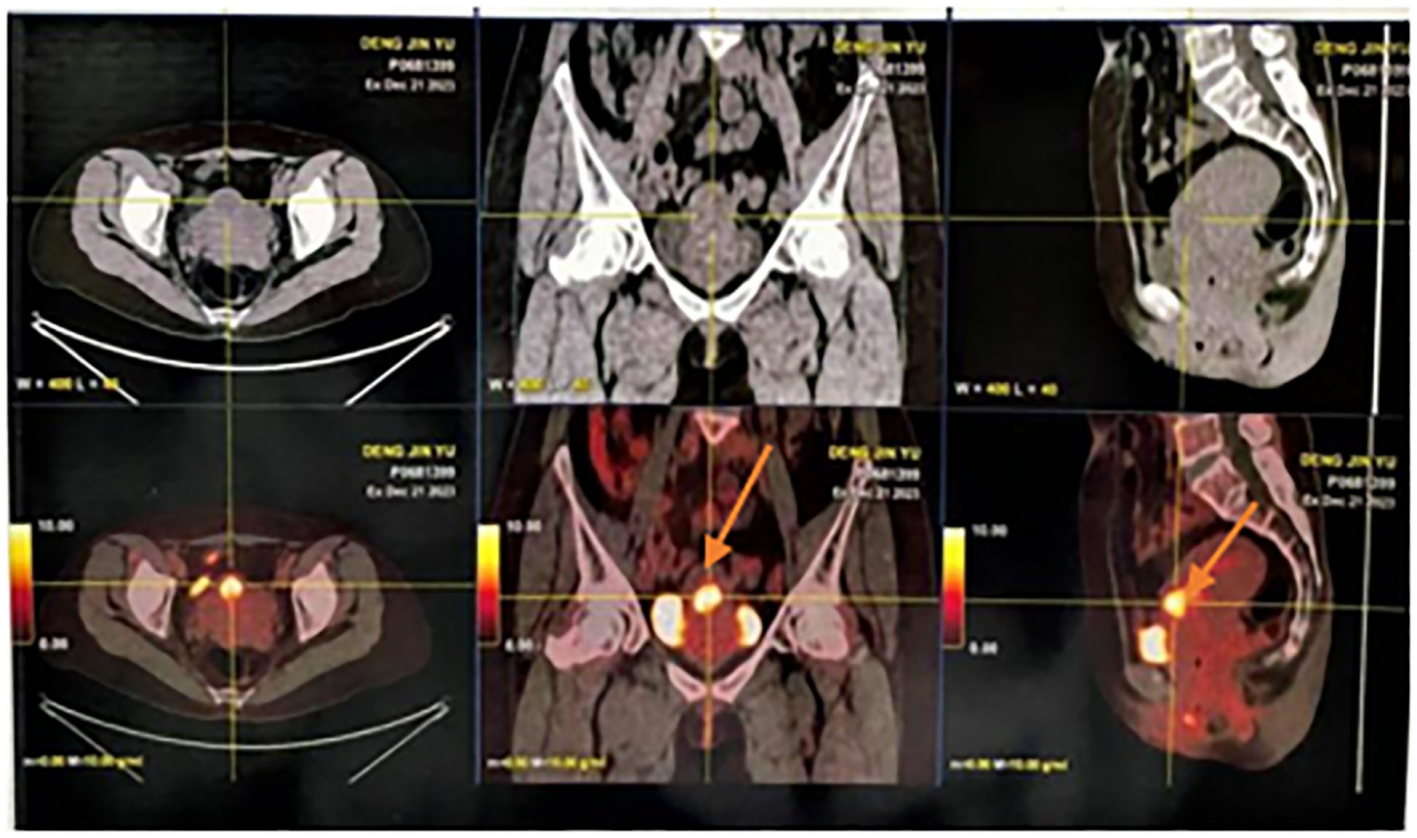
Whole body PET/CT image.
